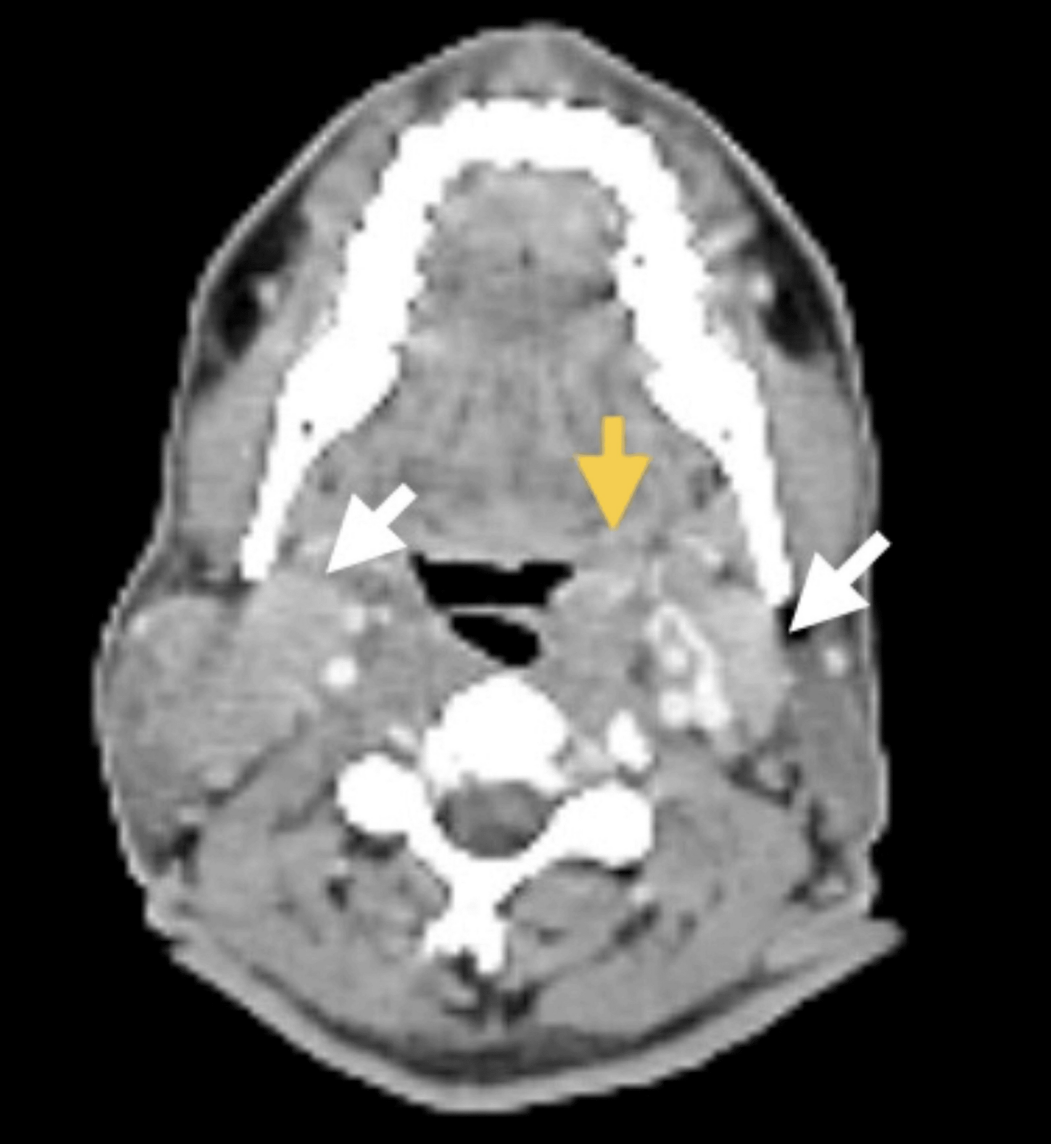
Axial contrast CT done prior to treatment. The imaging shows a fluorodeoxyglucose (FDG)-avid, enhancing lesion involving the base of the tongue, vallecula, and epiglottis on the left (yellow arrow), as well as CT-enhancing, enlarged bilateral level 2 cervical lymph nodes (white arrows).
